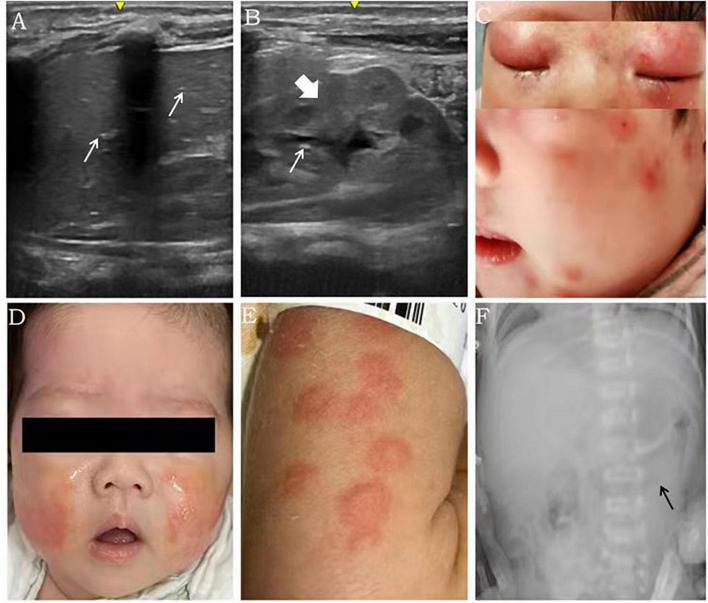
Imaging findings and rash features in patients with FPIES. Scattered echoes of gas in the anterior right lobe [(A) white arrow], diffuse bowel wall thickening (with a thickness of 3.3 mm) [(B), coarse arrow] and scattered echoes of gas in parts of the intestinal wall [(B), white arrow] were detected using ultrasound in case 1. Numerous red exudative papules fused and distributed all over the body, including the face, were observed in case 2 (C). Both the upper and lower eyelids were red and swollen with little ulceration (C). The rash in case 2 improved gradually after avoiding milk ingestion (D). Urticaria was distributed all over the body in case 3 (E). Distended loops of intestine were seen in X-ray in case 4 [(F), black arrow)].
